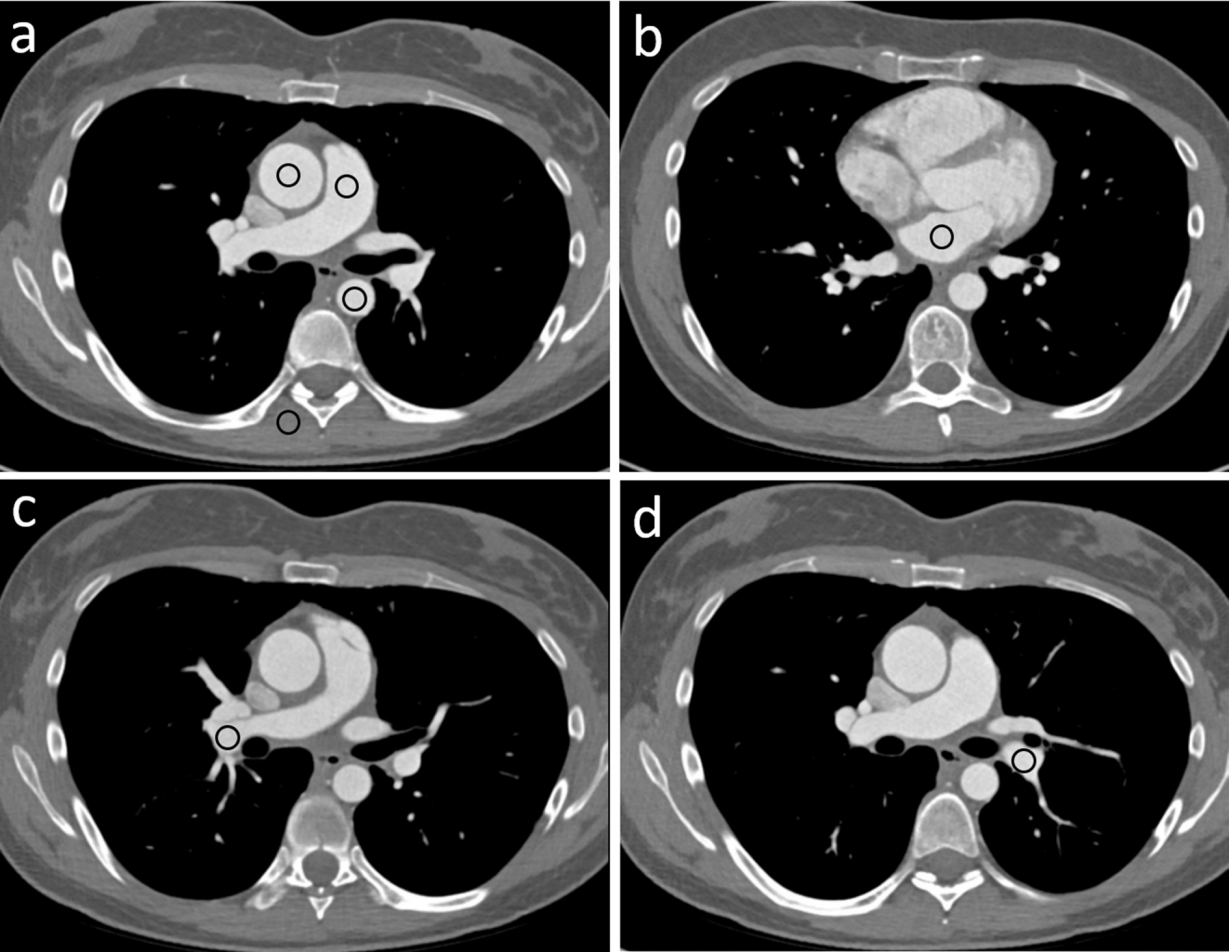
Measurements with ROIs in enhanced axial chest CT scans of the (a) pulmonary artery, ascending aorta, descending aorta, and paravertebral muscle; (b) left atrium; (c) right pulmonary artery; and (d) left pulmonary artery. ROI, region of interest.
